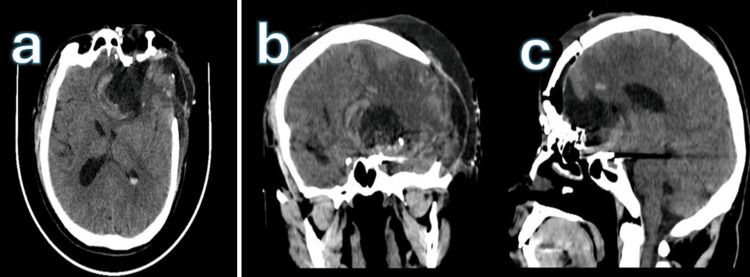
Postoperative non-contrast head CT scan, 2024 Axial (a), coronal (b), and sagittal (c) sections showing the left orbitozygomatic craniectomy site, with a small residual hyperdense lesion at the left anterior skull base and a hypodense area consistent with the surgical bed.
